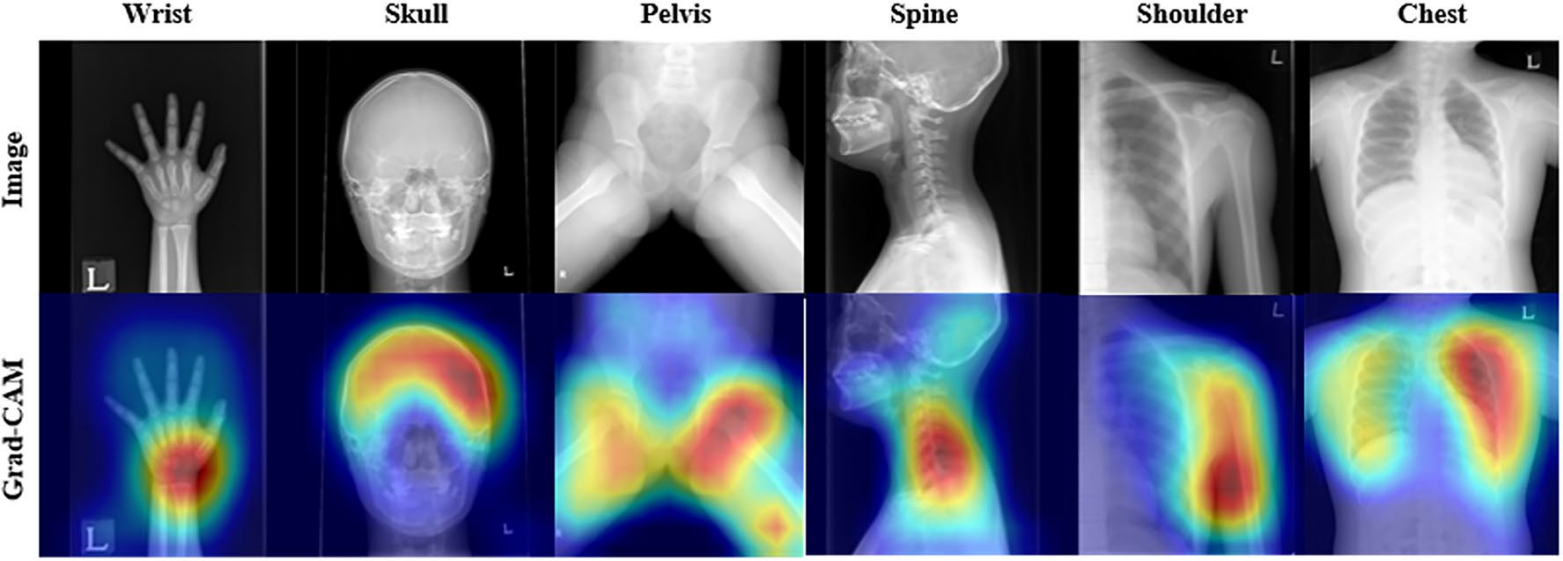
Plots of Model-PedXnet-7C model’s Grad-CAM activation maps of radiographic views recognition task as an upstream task with independent validation sets according to each labeling method. *Grad-CAM* gradient-weighted class activation mapping.
